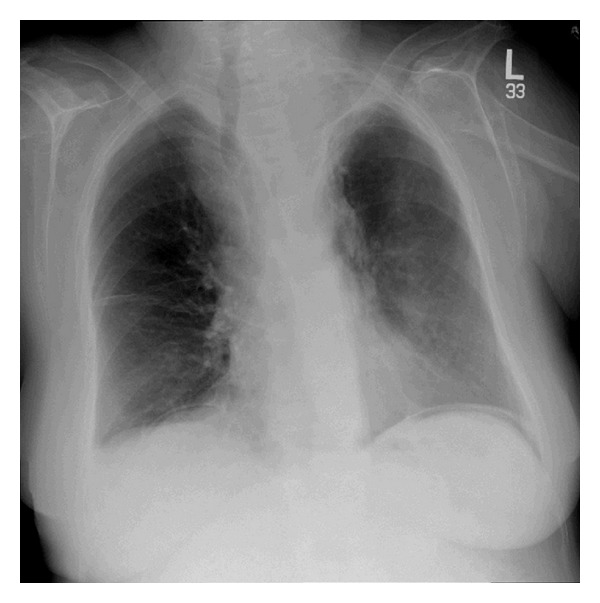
Upright chest plain radiograph (X-ray) showing free air (gas) under diaphragm.
